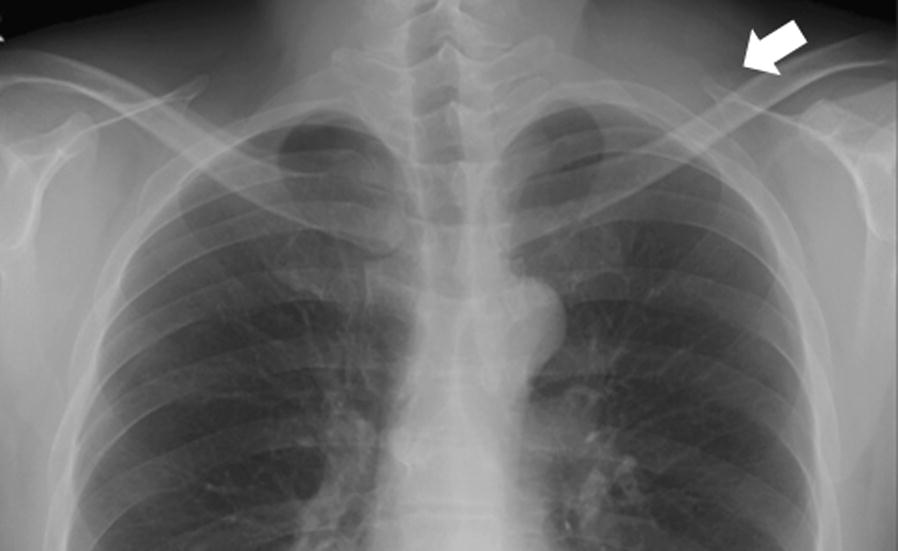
The radiographic finding show a soft tissue mass on the left neck. There is no calcification in the mass
